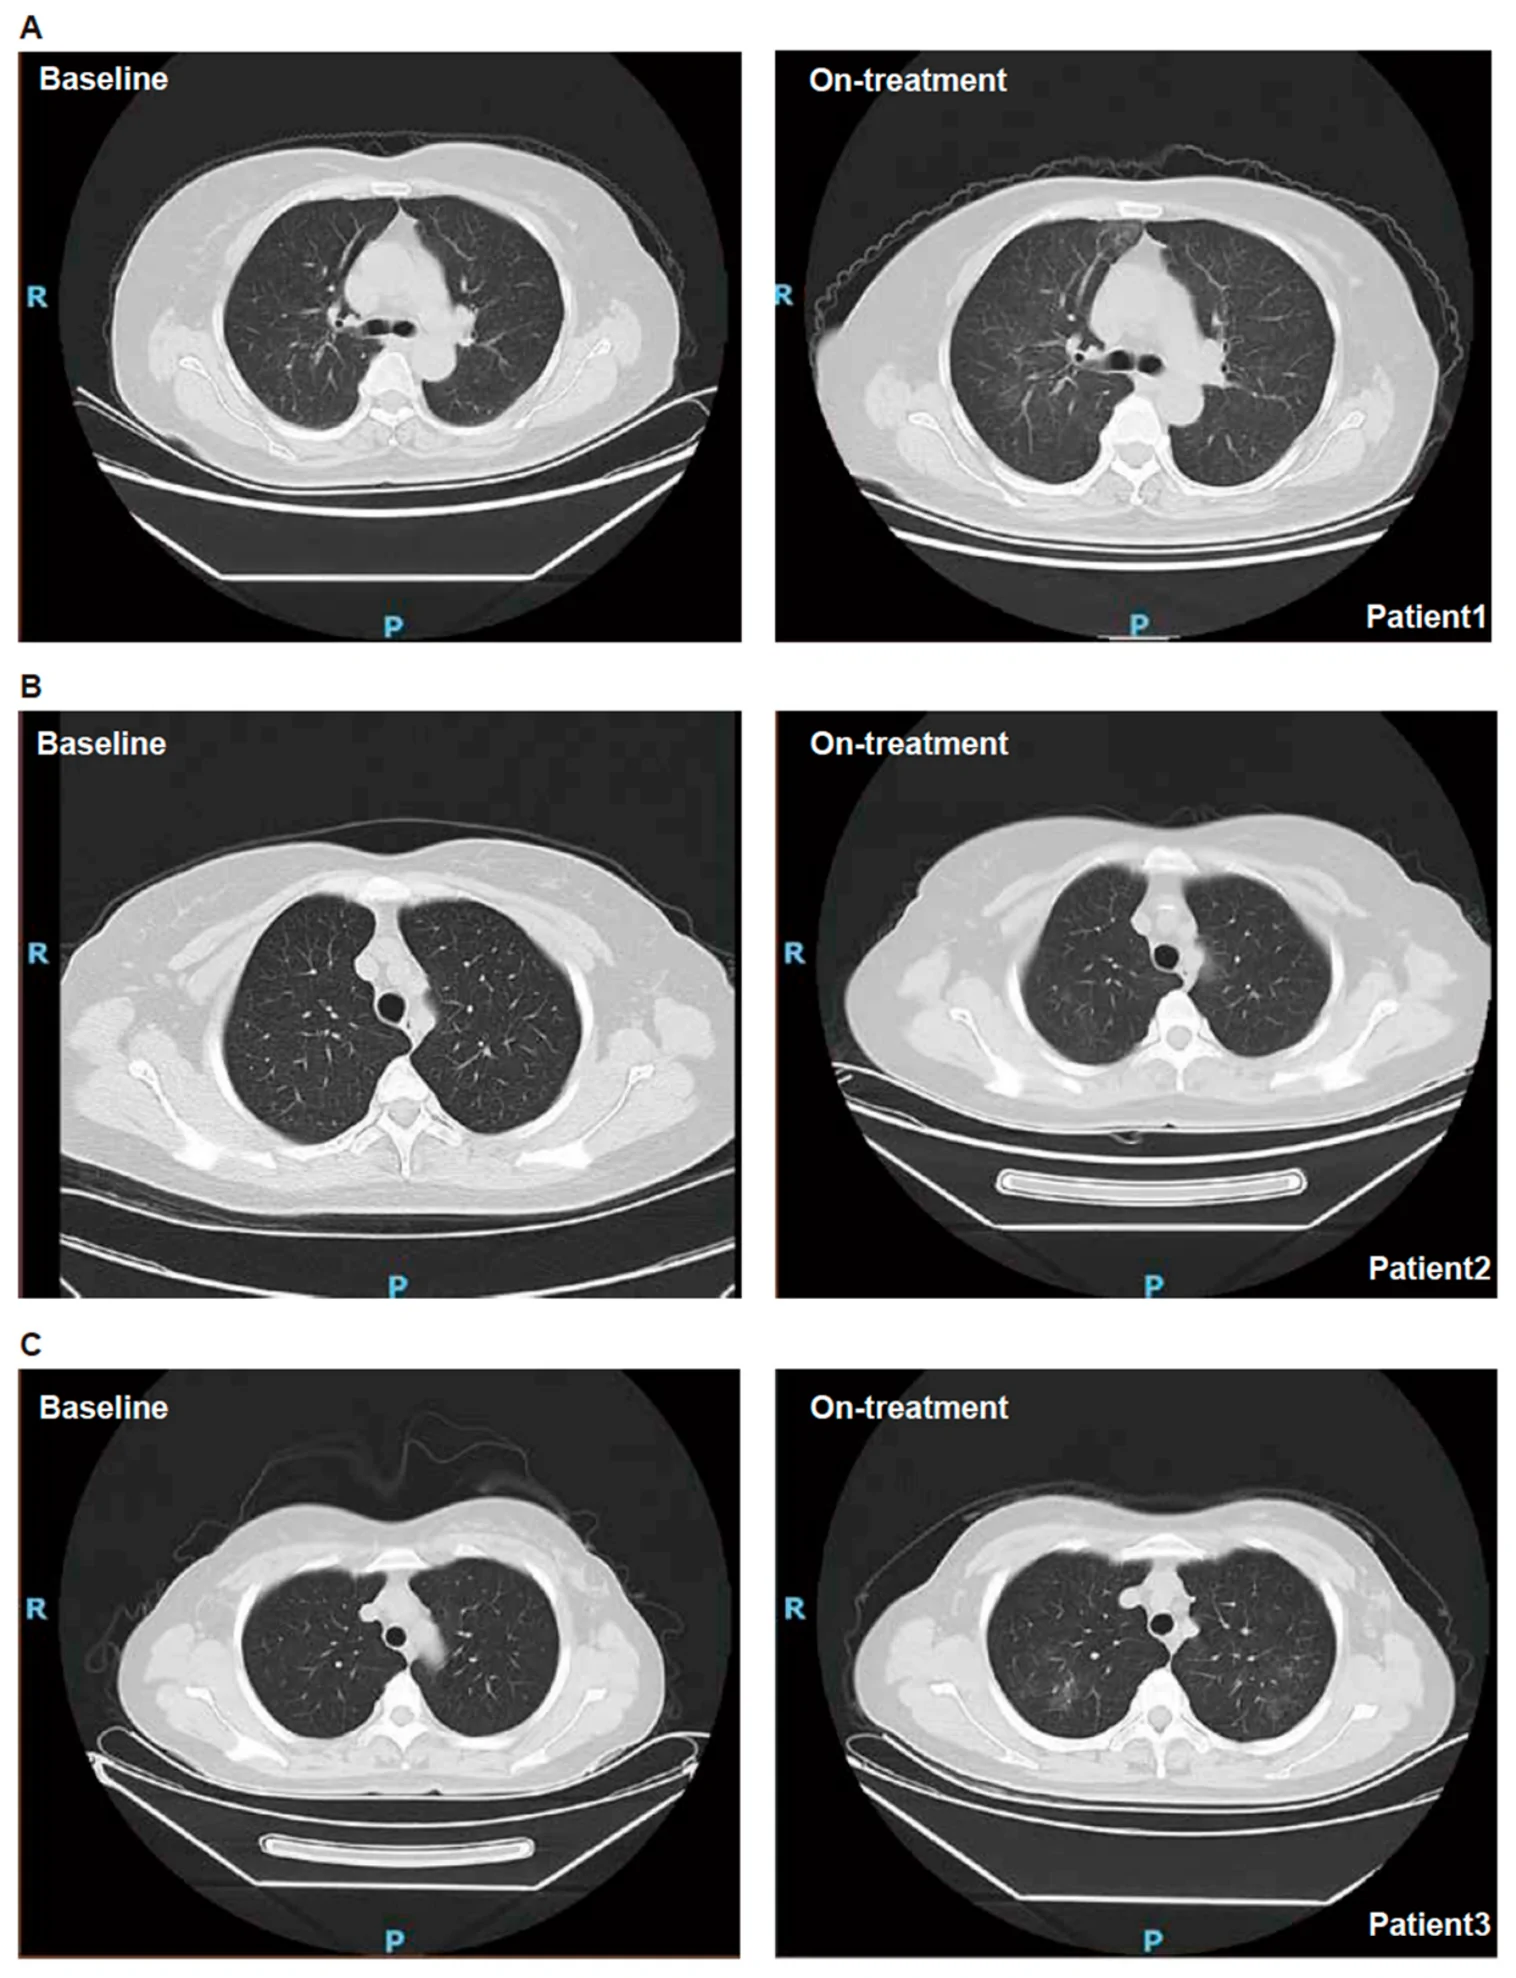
Representative paired baseline and on-treatment CT images. (A) Ground-glass opacity and linear/strand-like opacity; (B) ground-glass opacity; (C) patchy opacity and ground-glass opacity. R, right; P, posterior.
